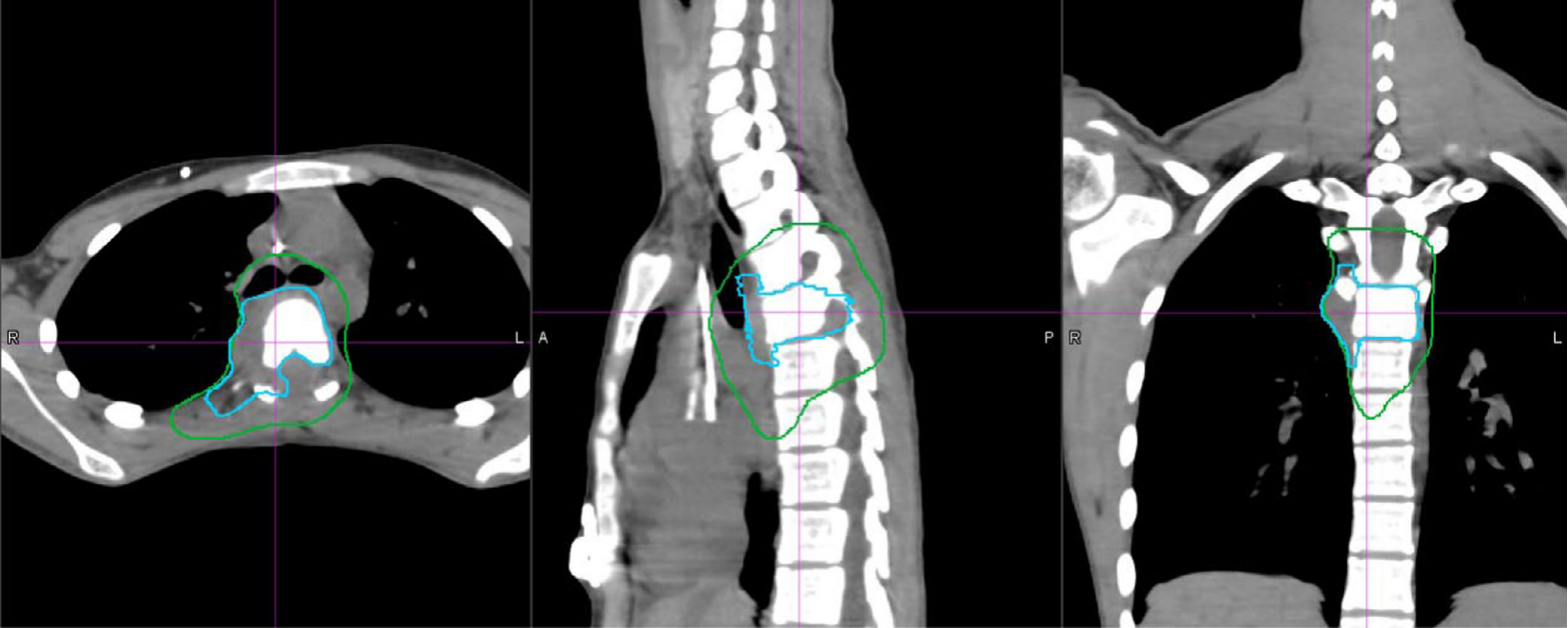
CT axial, sagittal, and coronal views of a patient with chest wall Ewing sarcoma at the region of T5. The green‐colored contour is CTV1 and the blue‐colored contour is CTV2.
